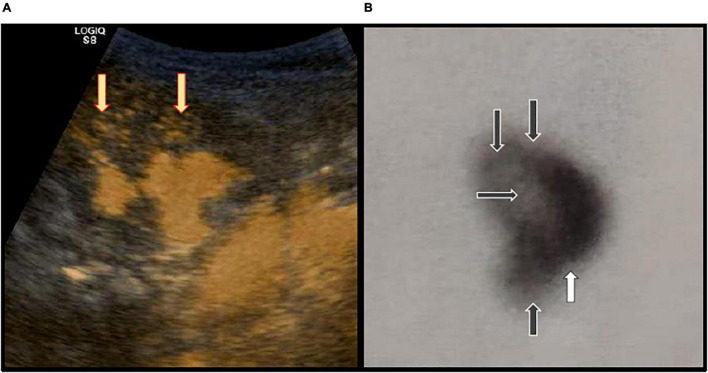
(A) Contrast-enhanced voiding urosonography (ceVUS) of the right kidney. The intrarenal reflux (IRR) is visible in the upper and middle renal segments (indicated by yellow arrows). (B) DMSA scan of the right kidney shows reduced DMSA signals (indicated by black arrows) in the upper, middle, and lower renal segments (indicated by black arrows), and a scar in the lower renal segment (indicated by white arrow).
